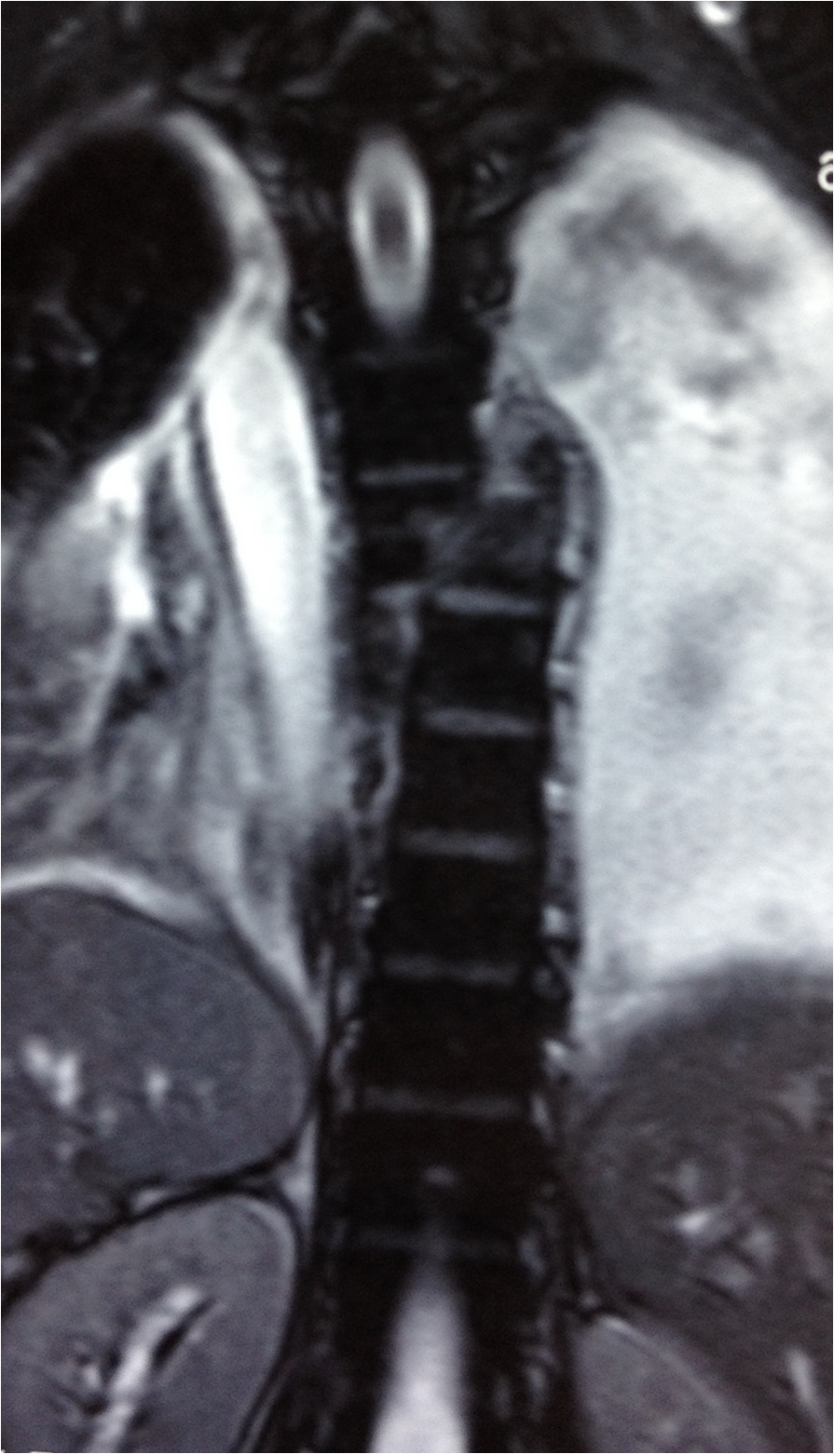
Magnetic resonance imaging showed spinal fracture-dislocation of T6 to T7.
